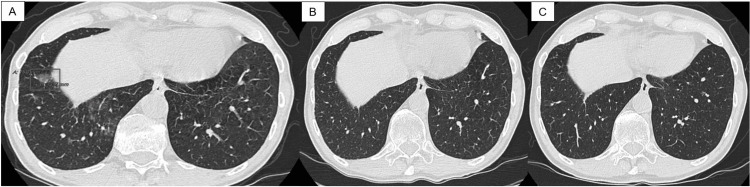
(A) Chest CT in January 2020 before the triple antibiotic therapy for the mycobacterial lung infection initiated; 15 mm × 12 mm ground glass nodule is outlined in the right lower lobe; (B) chest CT in August 2020 after three months of triple antibiotic therapy; (C) chest CT in February 2021 after nine months of discontinuing the triple antibiotic therapy CT: computerized tomography
